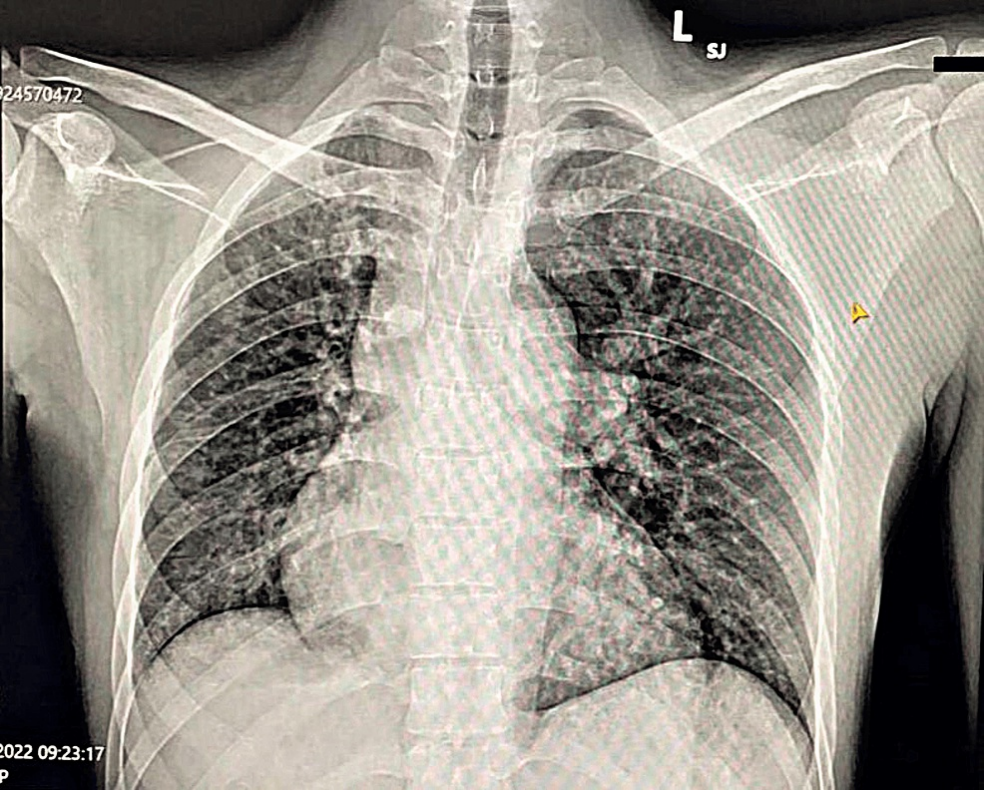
Chest X-ray, posteroanterior view Enlarged cardiac silhouette with prominent pulmonary trunk and pulmonary arteries proximally
